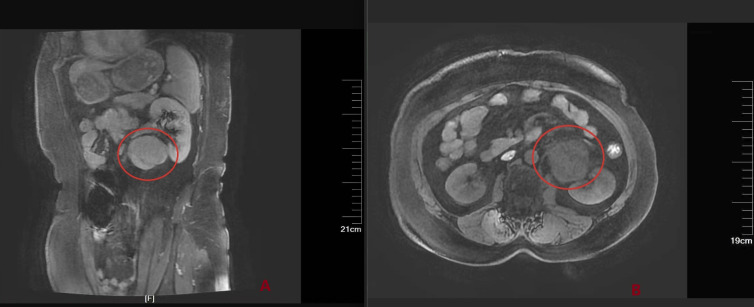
MRI: A circular mass can be seen in front of the left kidney in the retroperitoneal area, no 182 invasion is observed. (A) Sagittal plane. (B) Transverse plane. Before the surgery, we performed ultrasound−guided percutaneous needle biopsy on the patient. As shown in Figure 2. The ultrasound−guided percutaneous needle biopsy result showed CD21(+), CD79a(+), CD20(+), CD34(+), CD38(+), and CD138(+), suggested Unicentric Castleman Disease.
